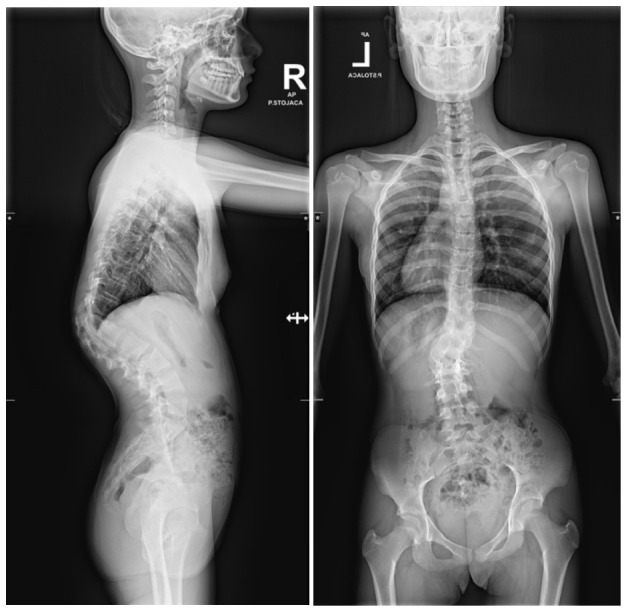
Standing posteroanterior and lateral full-spine radiography showed a congenital vertebral anomaly, consisting of a lower-thoracic (T11 level), leading to a progressive structural kyphoscoliotic deformity.
